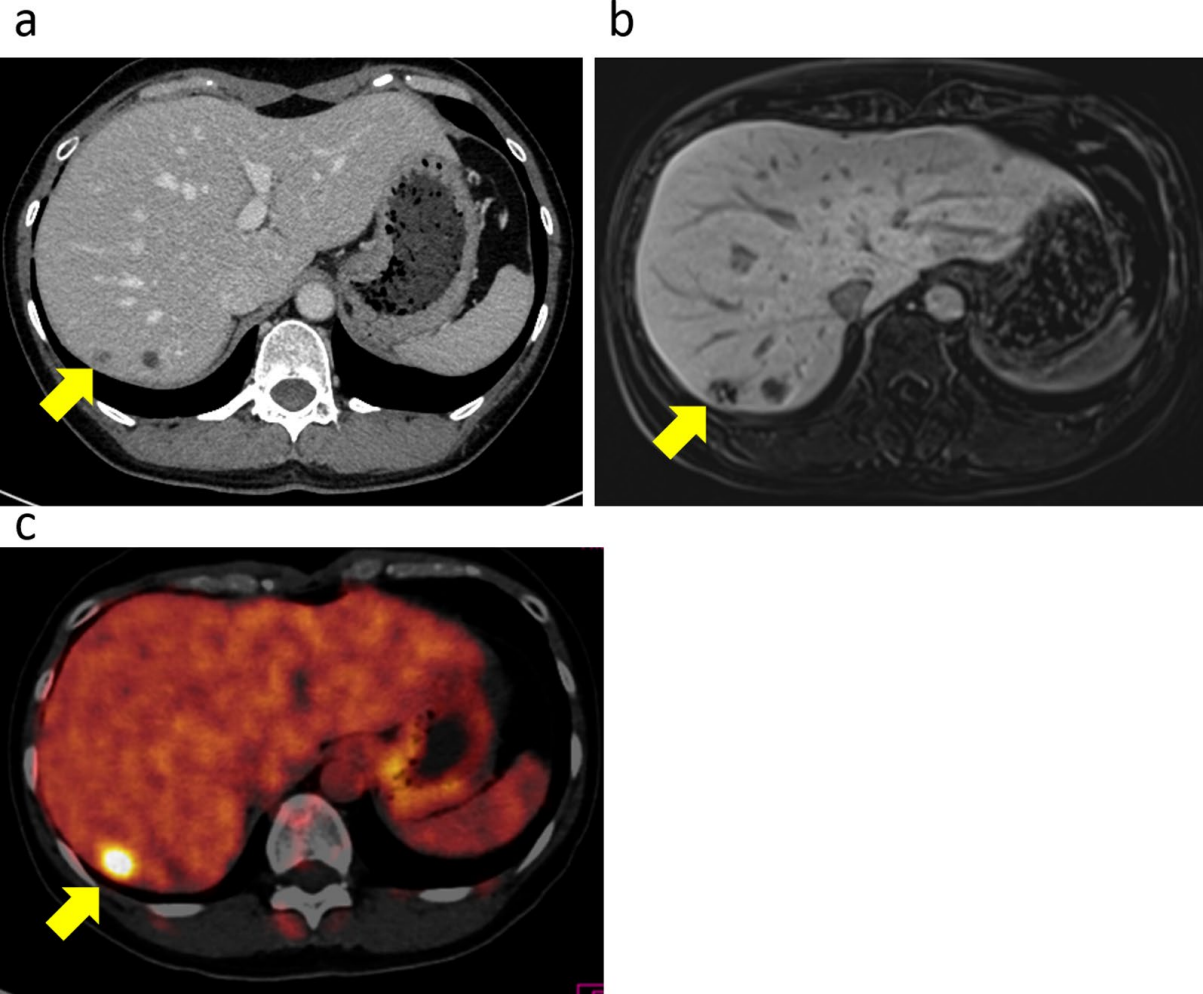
Imaging findings of the liver metastasis. a CT demonstrated a 14-mm-sized tumor with a faint contrast effect on the margin of liver S7. b The tumor showed a low signal intensity in the hepatocellular phase of Gd-EOB-DTPA-enhanced MRI. c The tumor showed strong accumulation of FDG on PET–CT
